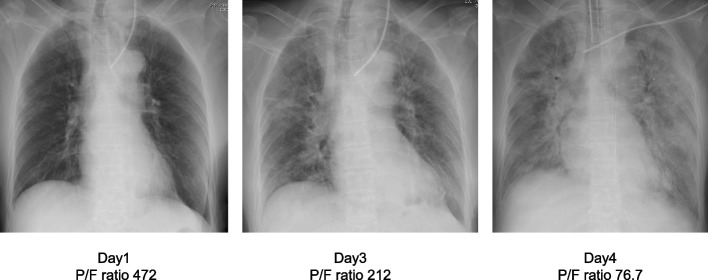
Chest radiograph, P/F ratio = PaO_2_/FiO_2_ ratio
